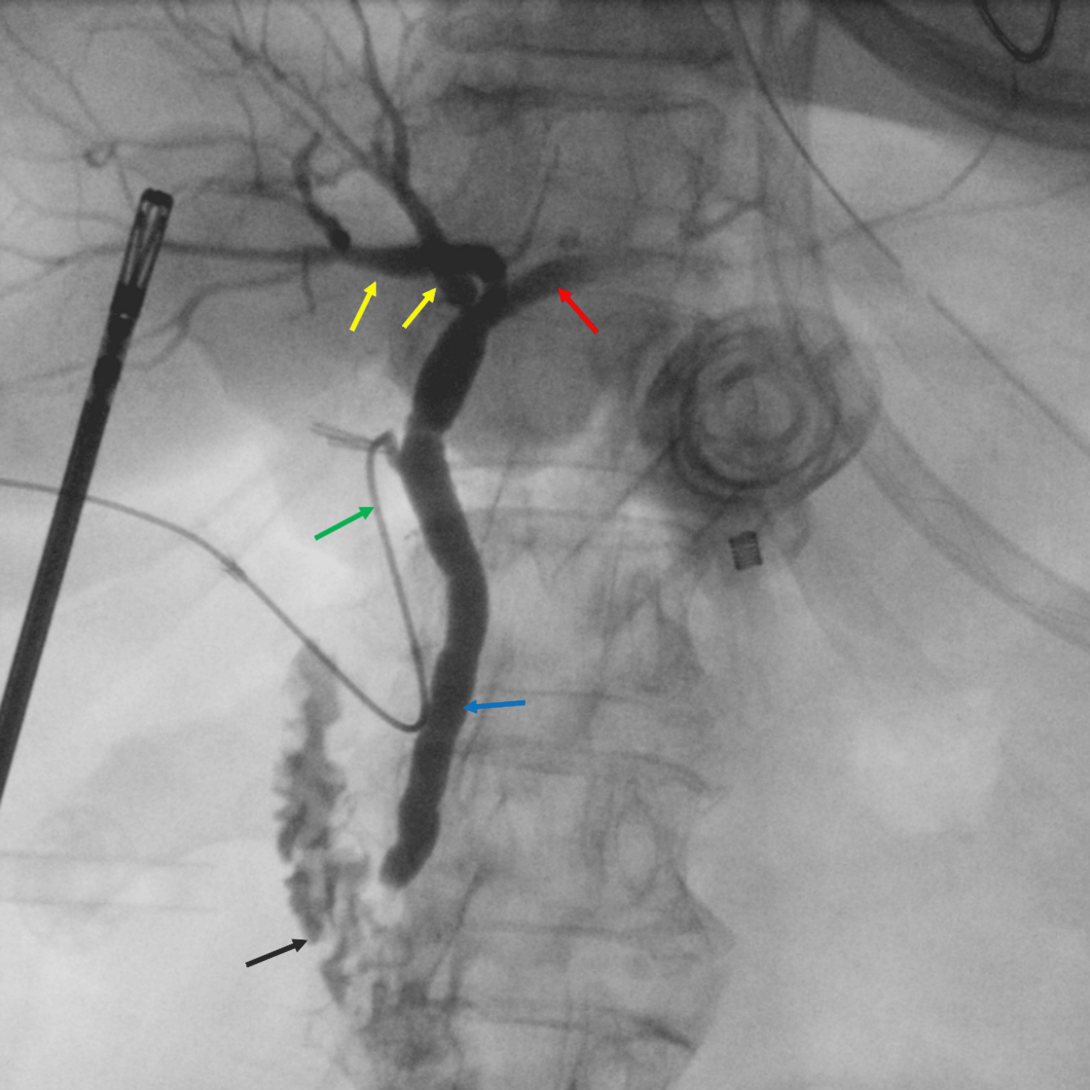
Intraoperative cholangiogram X-ray shows fluoroscopic contrast injection through an angiocatheter (green arrow), demonstrating full opacification of the cystic duct (red arrow), common bile duct (blue arrow), and both hepatic ducts (yellow arrows), with clear flow into the duodenum (black arrow) and no signs of ductal injury.
